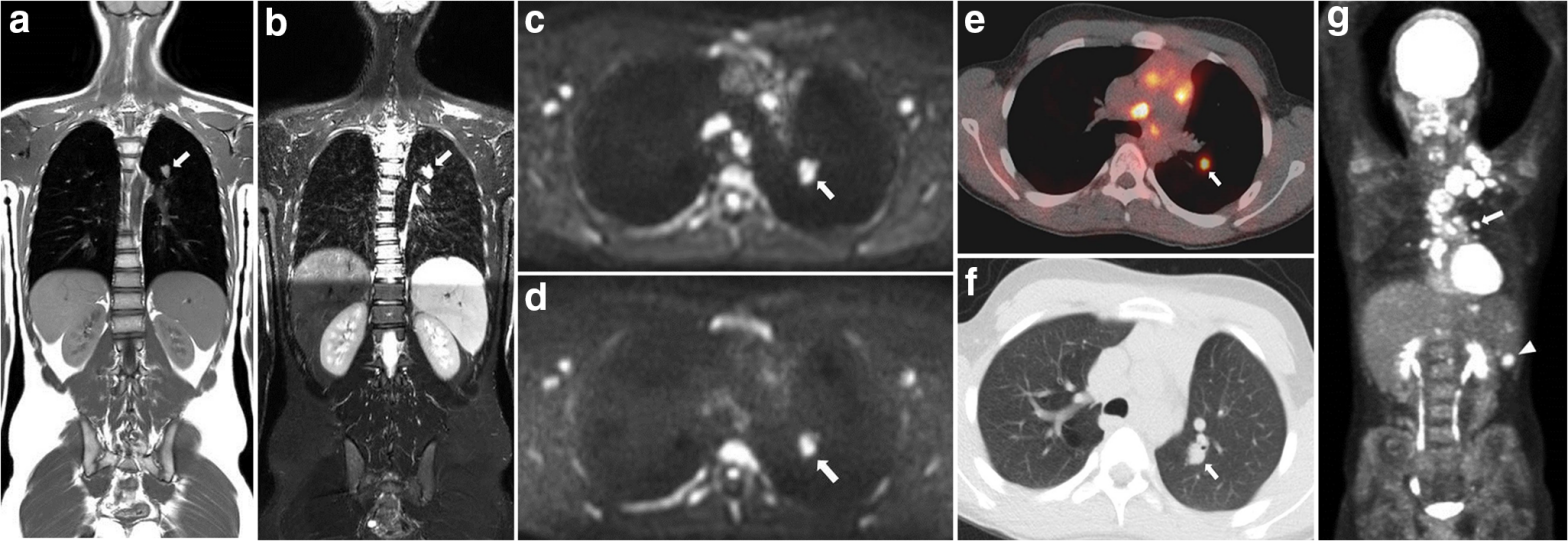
A 15-year-old boy with stage IV Hodgkin lymphoma. Example of an interpretation error by the FDG-PET/CT reader. Involvement of the lung was misinterpreted as hilar involvement. Coronal T1-weighted (a) and T2-weighted (b) MRI show the involvement of the lung (arrows). At axial DWI (b100 (c) and b800 (d), DWI restricted diffusion is seen (arrows). At axial FDG-PET/CT (e) and CT (f), the lesion is seen as well (arrows). Coronal maximum intensity projection (MIP) of the FDG-PET/CT (g) shows involvement of the lung (arrow) and multiple lymph node stations including cervical, mediastinal, hilar and the spleen (arrowhead). Those were all seen at WB-MRI as well.
